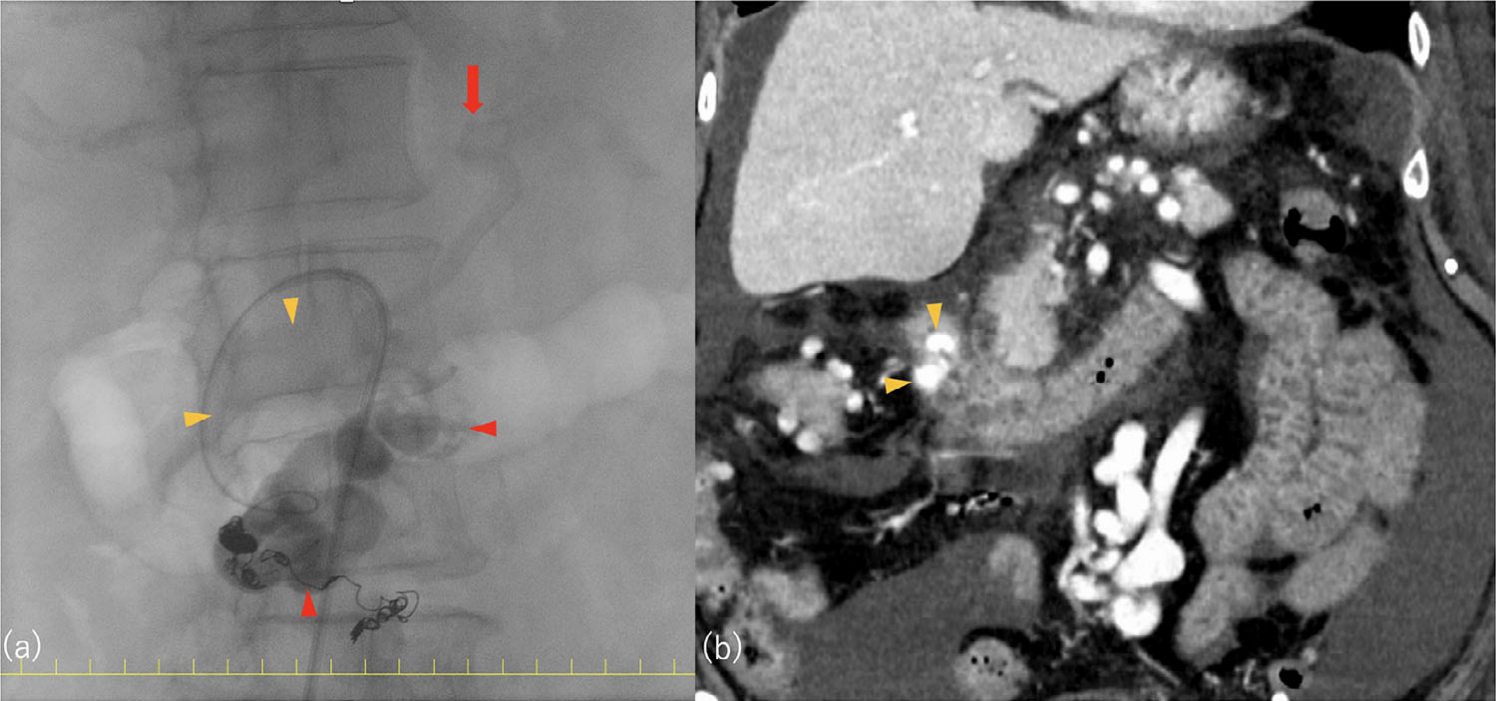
Partial obliteration of duodenal varices following balloon‐occluded retrograde transvenous obliteration (BRTO) that resulted in incomplete embolization of the distal superior mesenteric vein (SMV) feeder area. (a) Fluoroscopic images acquired during BRTO. (b) Contrast‐enhanced computed tomography (CT) image obtained 10 days after BRTO. Yellow arrowhead: region of duodenal varices not obliterated by BRTO. Red arrowhead: region of duodenal varices successfully obliterated by BRTO. Red arrow: feeder vein originating from the proximal superior mesenteric vein.
